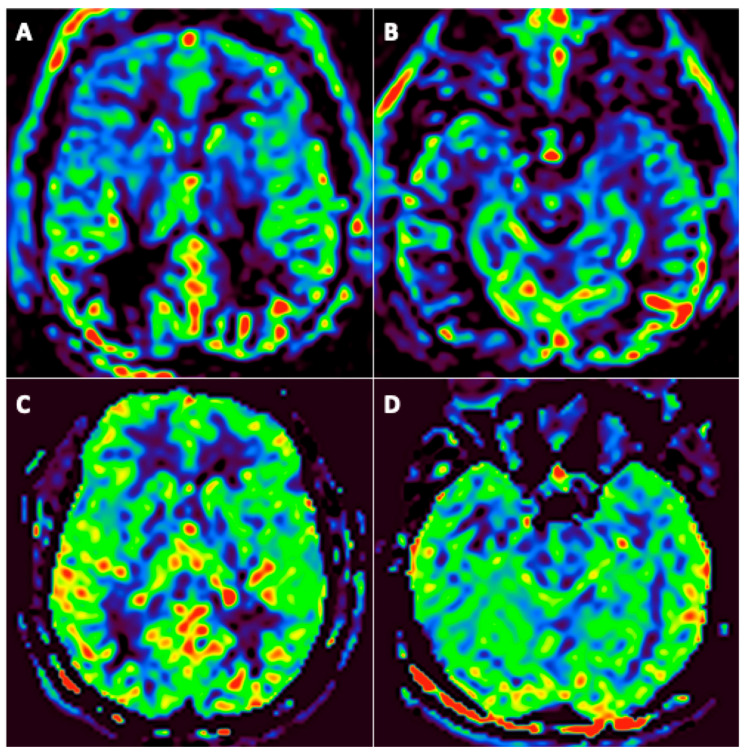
A 46-year-old man (#6) initially hospitalized in the ICU (A,B), who underwent a second MRI during follow-up 92 days later (C,D). Axial arterial spin labeling (ASL) brain perfusion imaging (A–D). Abnormal brain perfusion on initial imaging with frontotemporal hypoperfusion (A,B) and normalization during follow-up (C,D).
